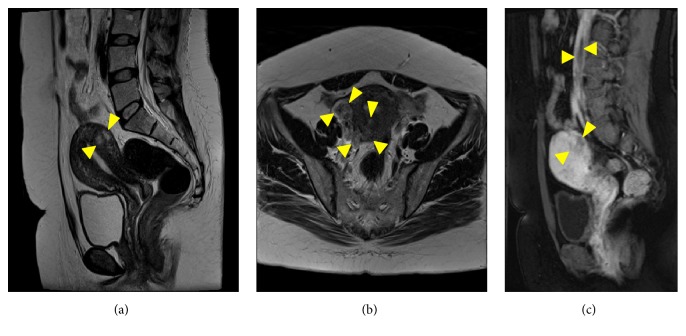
Magnetic resonance imaging (MRI): (a) on T2 weighted image, an irregular tumor is identified in the right posterior wall of the uterus, which exhibited heterogeneous high signal intensity (arrowheads); (b) the right uterine vein and ovarian vein are dilated, and low and high intensity masses are depicted in the veins and originated from the high intensity tumor in the posterior uterine wall (arrowheads); (c) sagittal view of dynamic contrast-enhanced MRI demonstrates large filling defect in the inferior vena cava with gradually increasing enhancement (upper arrowheads) and poor enhancement of tumor in the posterior uterine wall (lower arrowheads).
